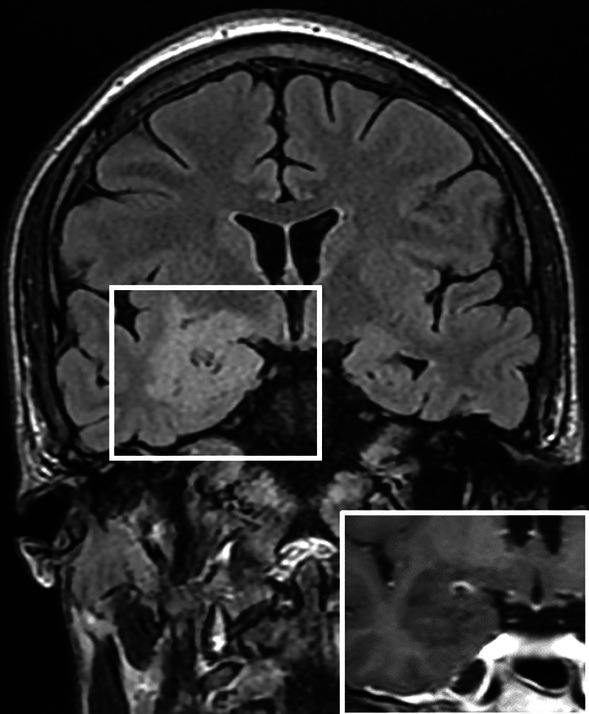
Coronal T2‐weighted FLAIR imaging demonstrates a hyperintense right medial temporal lobe mass that extends into the inferior right frontal lobe and bulges medially into the incisura. Gadolinium‐enhanced coronal T1‐weighted imaging shows the mass elevating the right middle cerebral artery but no significant contrast enhancement within the tumor (inset).
